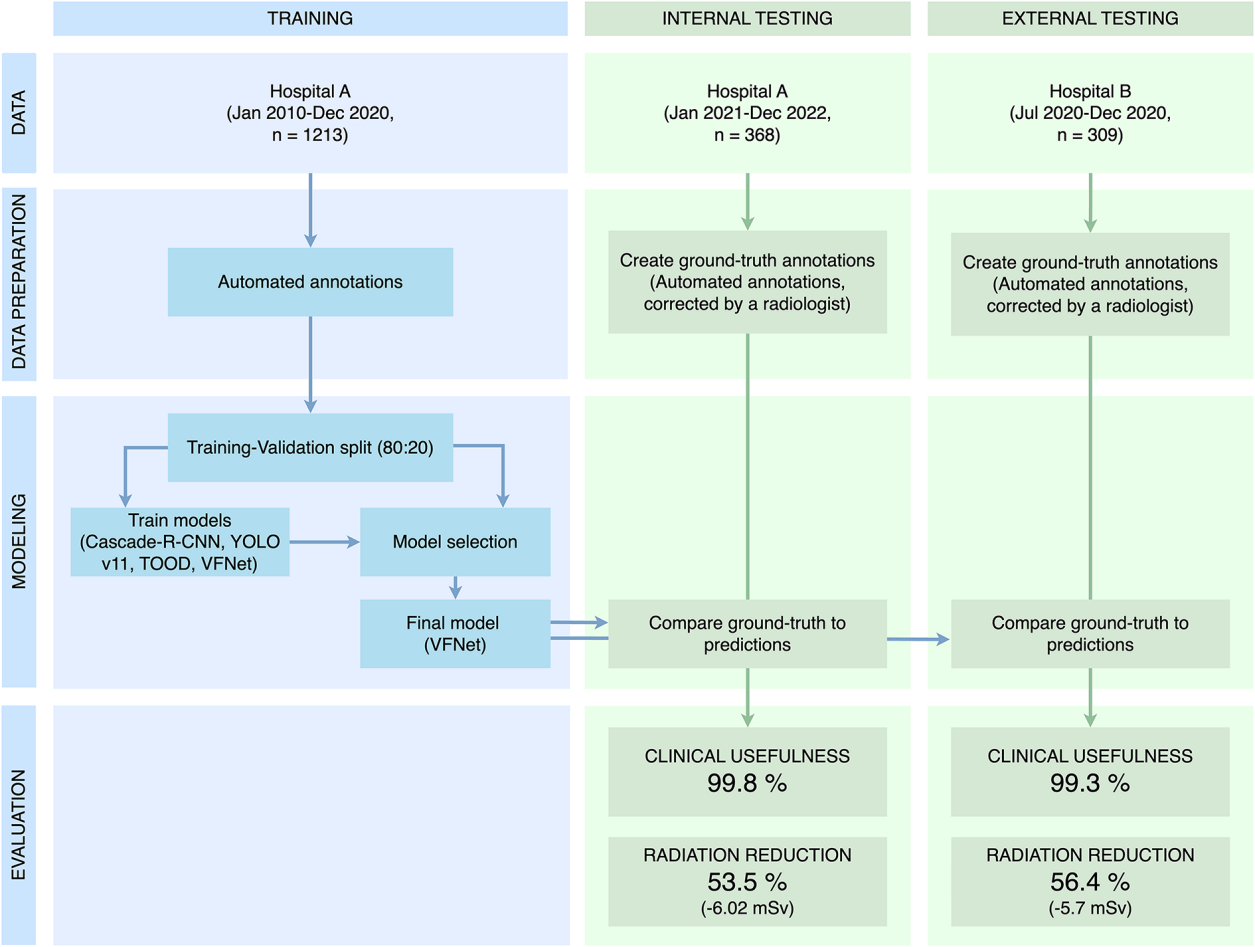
Study design. The aim of this study was to develop a network capable of delimiting the LAA in CT localizers, thereby automating the task usually performed by technologists. We used three independent cohorts from two hospitals. Due to the variation in the shape of the LAA, annotations from the CT scan were transferred back to the localizer. For model development, three networks were validated using a simple 80:20 split. The best-performing model in terms of the Dice coefficient was selected and tested in two independent cohorts. The model was then evaluated for clinical utility (or accuracy) and radiation dose compared to whole-heart scanning. *LAA* left atrial appendage, *TOOD* task-aligned one-stage object detection, *VFNet* VariFocalNet.
